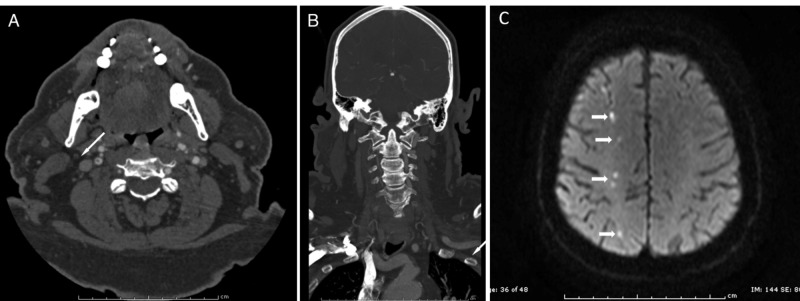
Computed tomographic angiogram and magnetic resonance imaging of Case 1 (A) Computed tomographic angiogram (CTA) of the neck, axial view, demonstrating the “target sign,” indicating intraluminal carotid thrombus and severe carotid artery stenosis (arrow). (B) Neck CTA, coronal view, demonstrating intraluminal brachiocephalic artery thrombus (arrow). (C) Brain magnetic resonance image demonstrating the watershed diffusion restriction pattern (white arrows).
